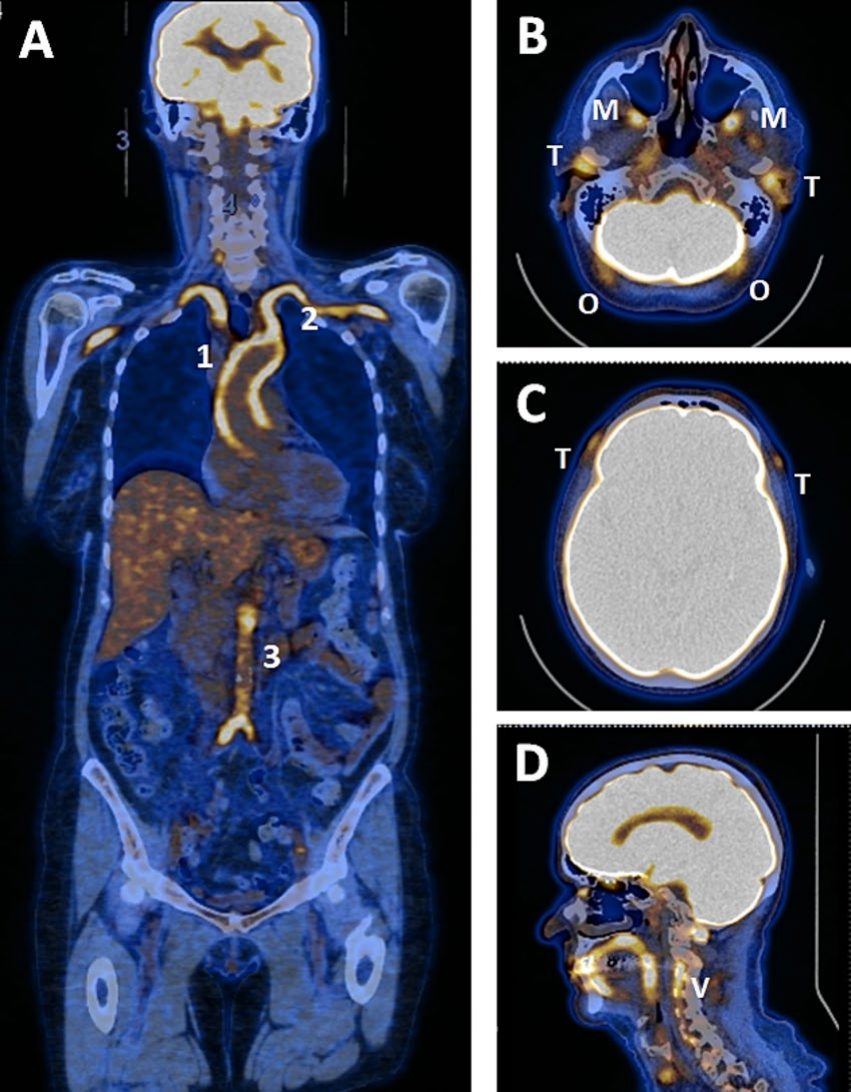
PET/CT study of a patient with GCA involving cephalic and large arteries. (A) study of large arteries showing grade 3 hypermetabolism of the ascending aorta (1), subclavian and axillary arteries (2) and from the abdominal aorta to the origin of the iliac arteries (3). (B–D) study of cephalic arteries showing significant hypermetabolism of the temporal (T), maxillary (M), occipital (O) and vertebral (V) arteries. (C) hypermetabolism of the frontal branch of the temporal artery (T).
